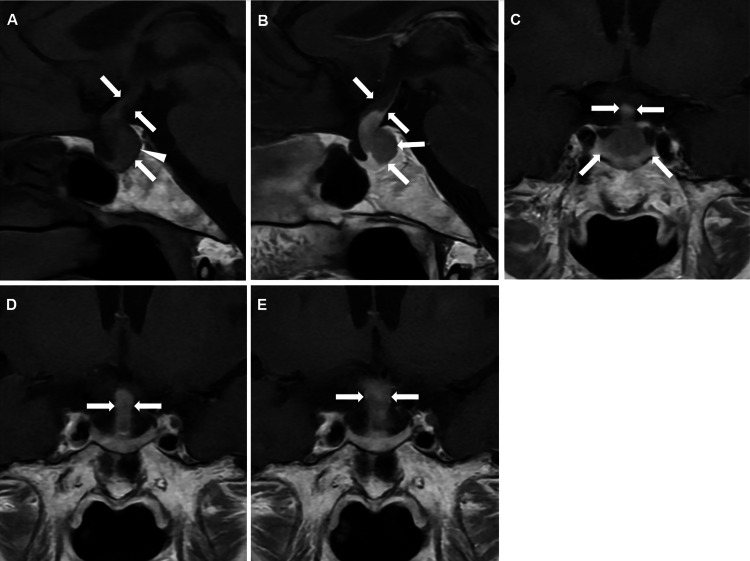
Pituitary magnetic resonance imaging (MRI) findings. MRI findings at presentation (A-D) and 234 days after the first admission (E). Non-contrast sagittal T1-weighted image (A), contrast-enhanced sagittal T1-weighted image (B), and contrast-enhanced coronal T1-weighted images (C-E). A mass-like lesion is seen extending from the pituitary gland to the pituitary stalk within the sella turcica. The normal high signal intensity of the posterior pituitary on T1-weighted imaging is absent (white arrows) (A). The pituitary stalk and posterior pituitary show homogeneous enhancement on the contrast-enhanced study, suggesting lymphocytic posterior hypophysitis (white arrows) (B). There is no evidence of compression of the optic chiasm by the pituitary gland (white arrows) (C). Compared with the initial MRI (white arrows) (D), further enlargement of the pituitary stalk is observed on the follow-up MRI (white arrows) (E).
